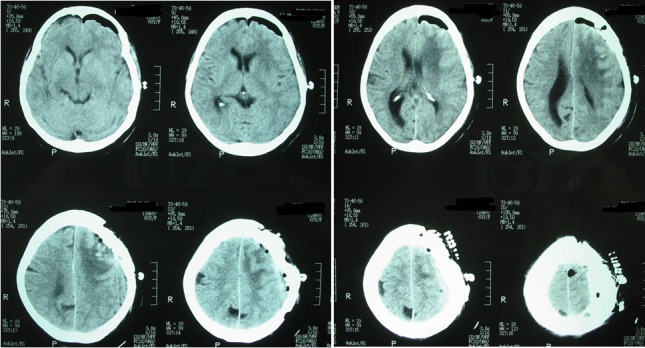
Postoperative brain CT scan Postoperative CT scan shows complete excision of the tumor with residual edema and pneumocephalus.
